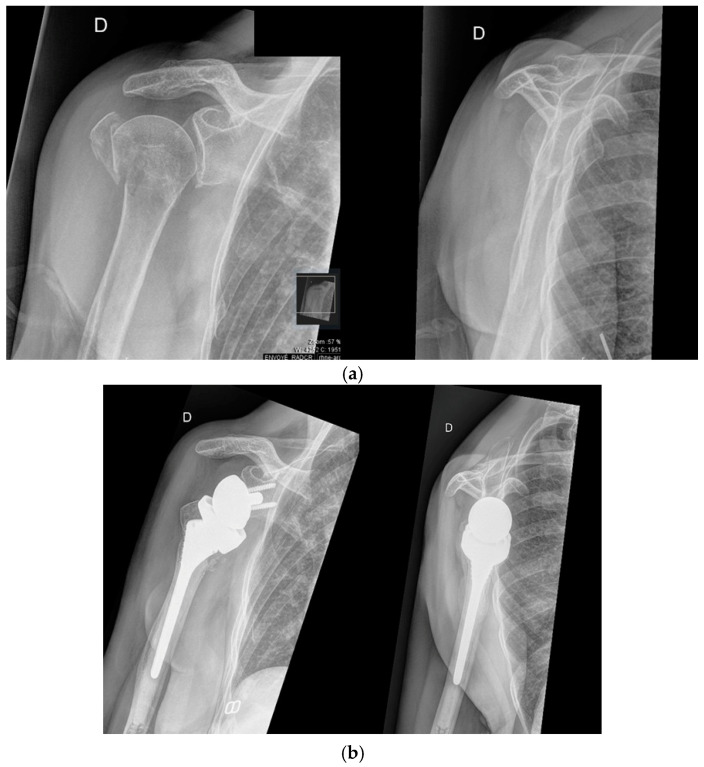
(a) In the figure, two radiographic projections: on the left, the anteroposterior projection and on the right, the Neer projection of a three-part fracture of the proximal humerus. (b) Treatment with reverse shoulder prosthesis of the fracture shown in figure (a), with anteroposterior projection on the left and Neer projection on the right.
